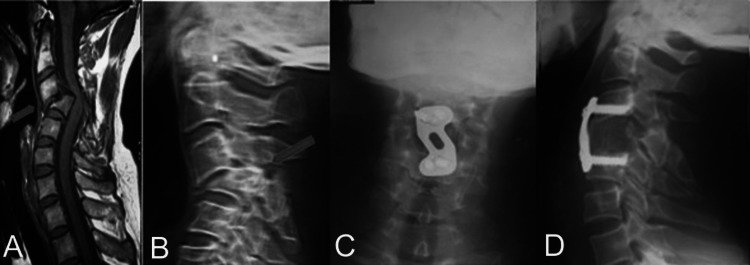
Anterior cervical corpectomy and fusion (ACCF) A: T1-weighted sagittal MR image of the cervical spine showing fracture-dislocation at C3/4 B: X-ray cervical spine in lateral view showing fracture-dislocation at C3/4 C: X-ray cervical spine in antero-posterior and (D) lateral view showing solid fusion mass at final follow-up
